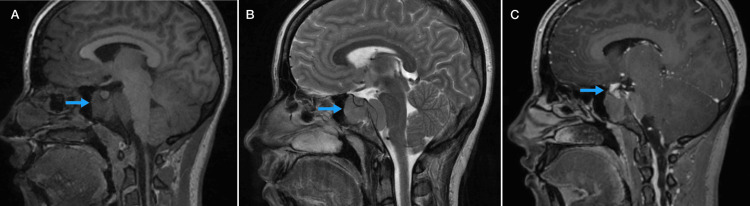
Magnetic resonance imaging (MRI) findings. (A) Sagittal T1-weighted MRI brain showing a homogeneous soft-tissue lesion arising from the sphenoid sinus with a hypo-intense signal (arrow). (B) Sagittal T2-weighted MRI brain showing a homogeneous soft-tissue lesion arising from the sphenoid sinus with a hypo-intense signal (arrow). (C) Sagittal T1-weighted post-contrast MRI brain demonstrating a soft-tissue lesion arising from the sphenoid sinus with diffuse restriction and mild enhancement (arrow).
